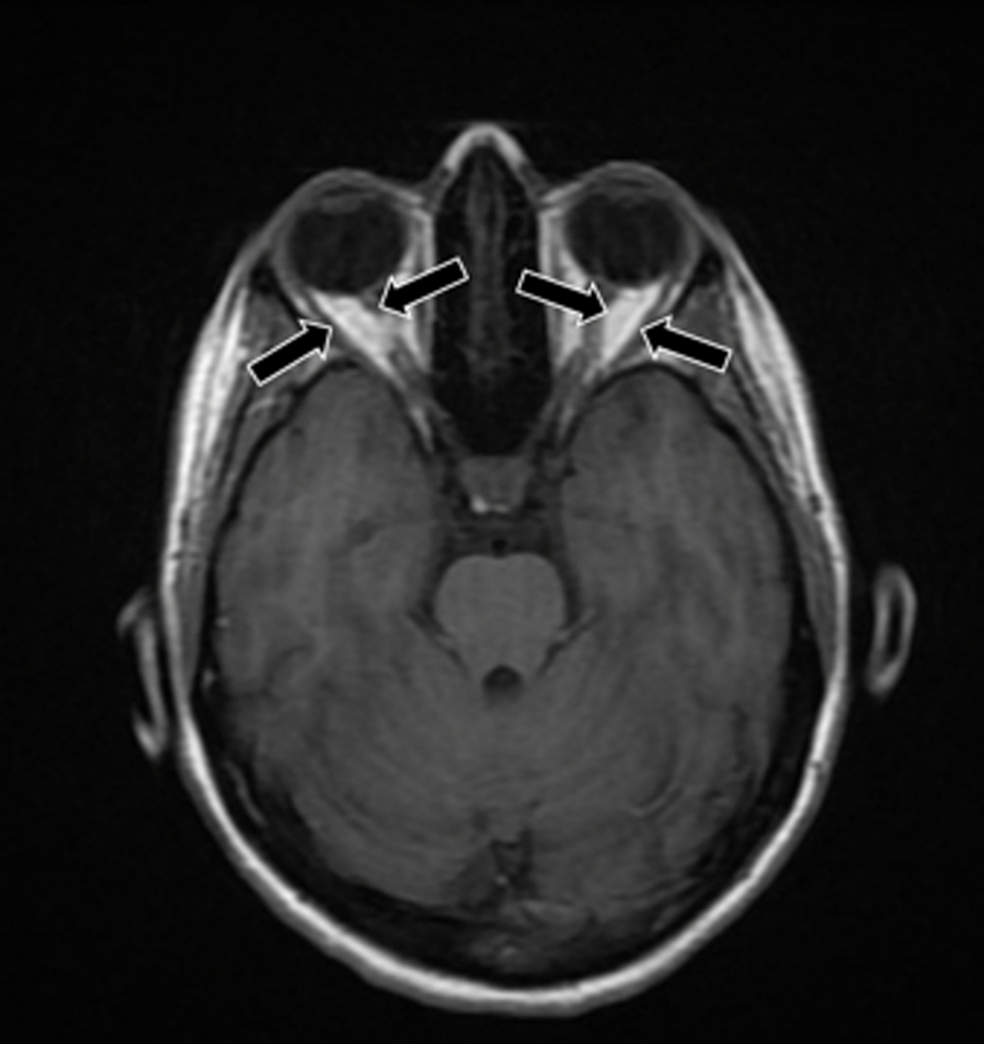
Brain CT revealing mild thickening of the bilateral optic nerves (black arrows).
